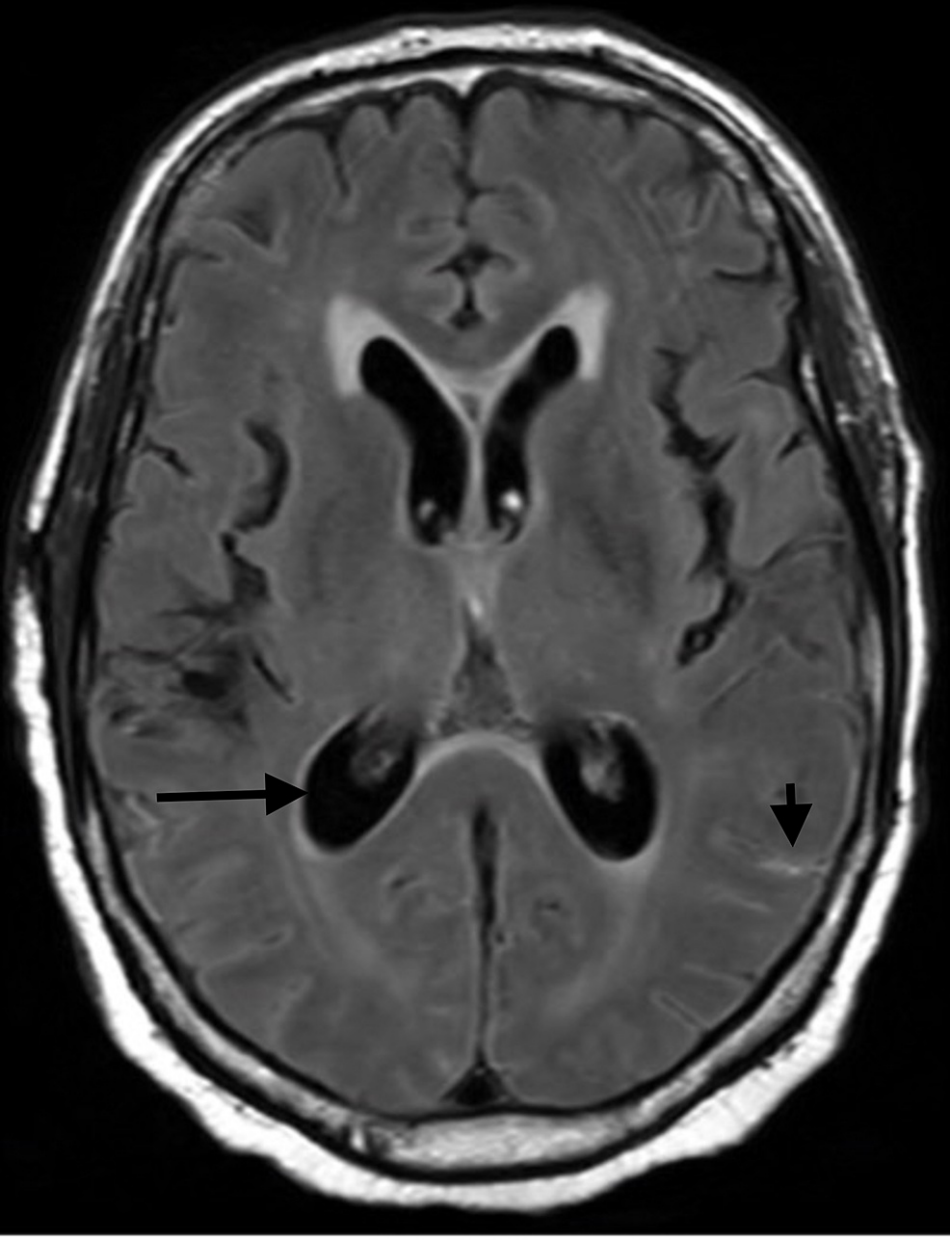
MRI of the brain on FLAIR sequences done three-weeks after admission The image is demonstrating new sulcal hyperintensities (short, black arrow) and hydrocephalus (long, black arrow) MRI: Magnetic Resonance Imaging, FLAIR: Fluid attenuated inversion recovery
